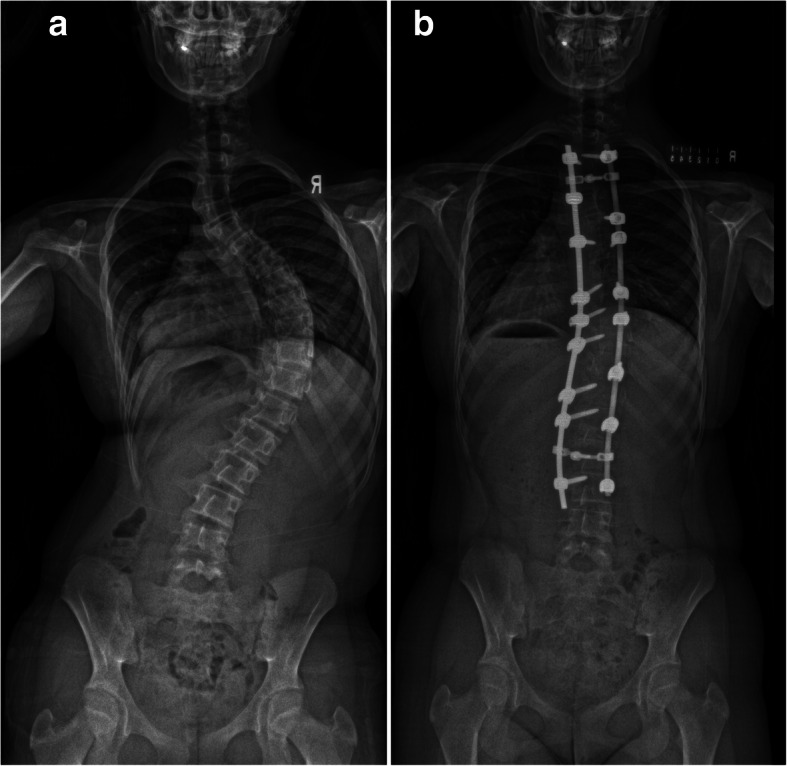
Pre-operative (a) and post-operative (b) radiographs of a 12-year-old girl with T2-L3 posterior spinal fusion for the Lenke 1 curve. Total operative time was 320 min with intra-operative blood loss of 900 ml
